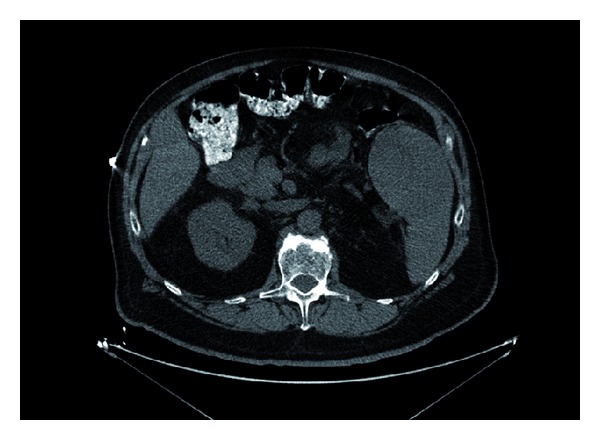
Abdominal CT showing splenic mass.
